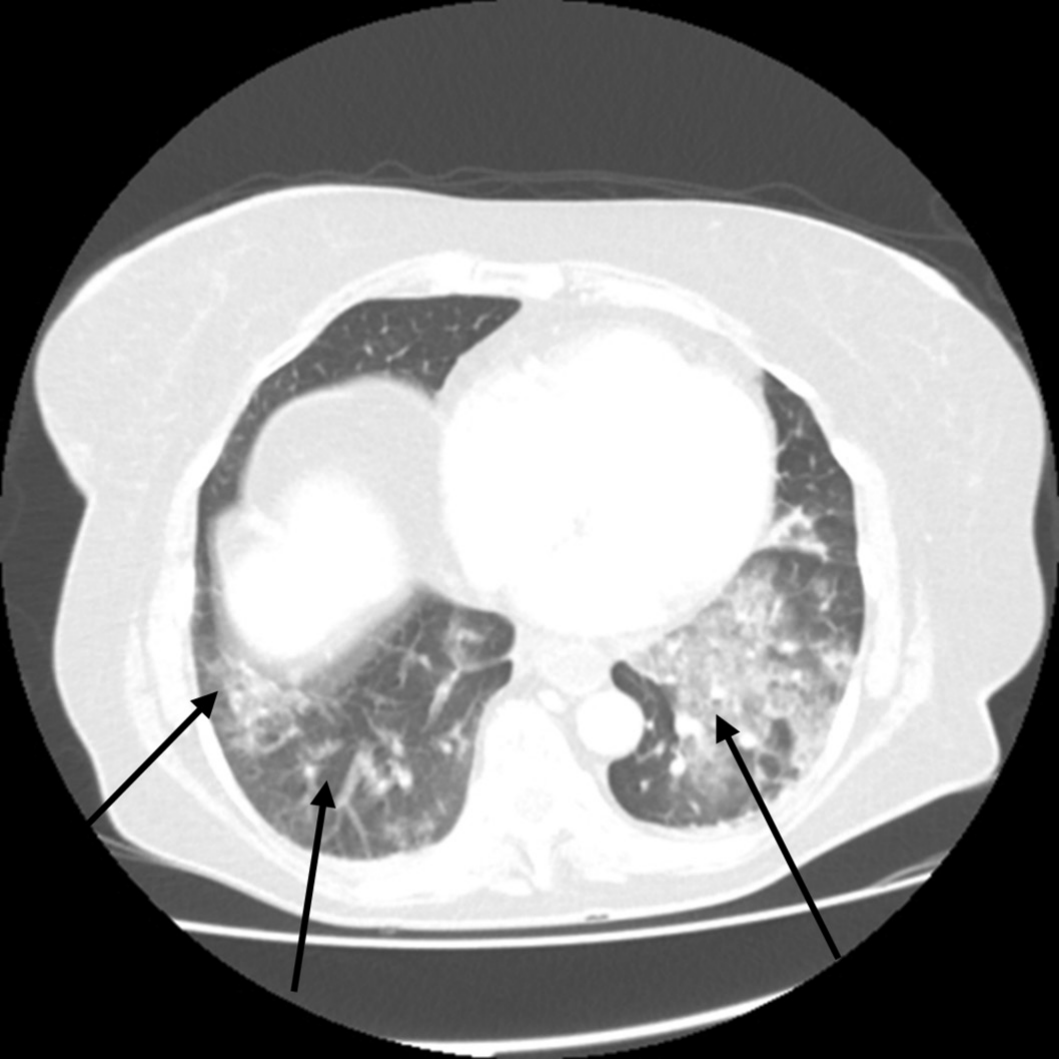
CT scan of the chest; Discrete and confluent ground glass opacities in bilateral visualized lower lobes, concerning for COVID pneumonitis. Minimal bilateral pleural effusion, 26/01/2021.
